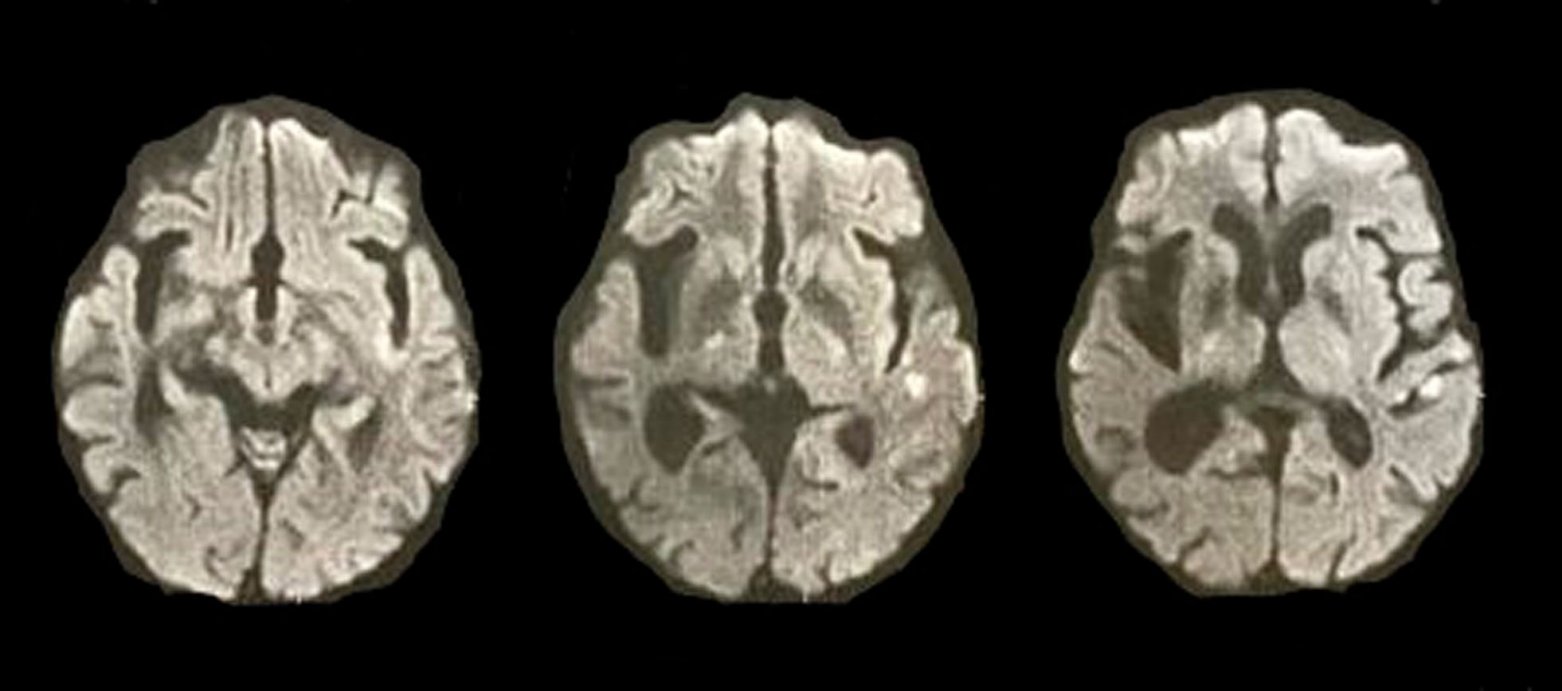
Follow-up 24 h magnetic resonance imaging diffusion film showing restricted vascular lesion along left insula and cortical auditory center
